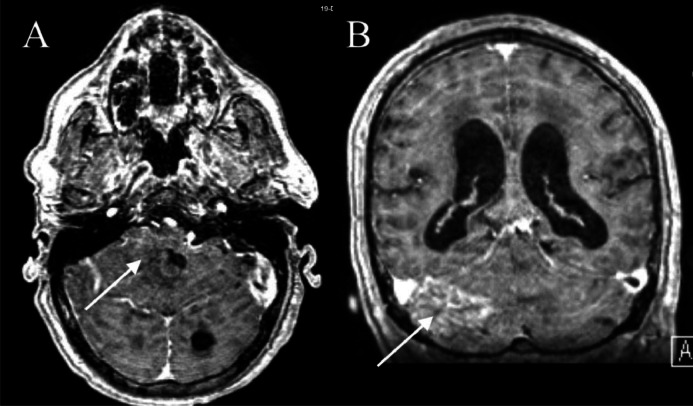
T1-weighted postcontrast MRI brain showed heterogeneous enhancement (arrows) within the area of the precontrast hyperintensity in the right cerebellum, seen on axial (A) and coronal (B) imaging. No discrete lesion was visualized. In retrospect, prominent vessels along the tentorium may have been indicative of an underlying vascular lesion.
